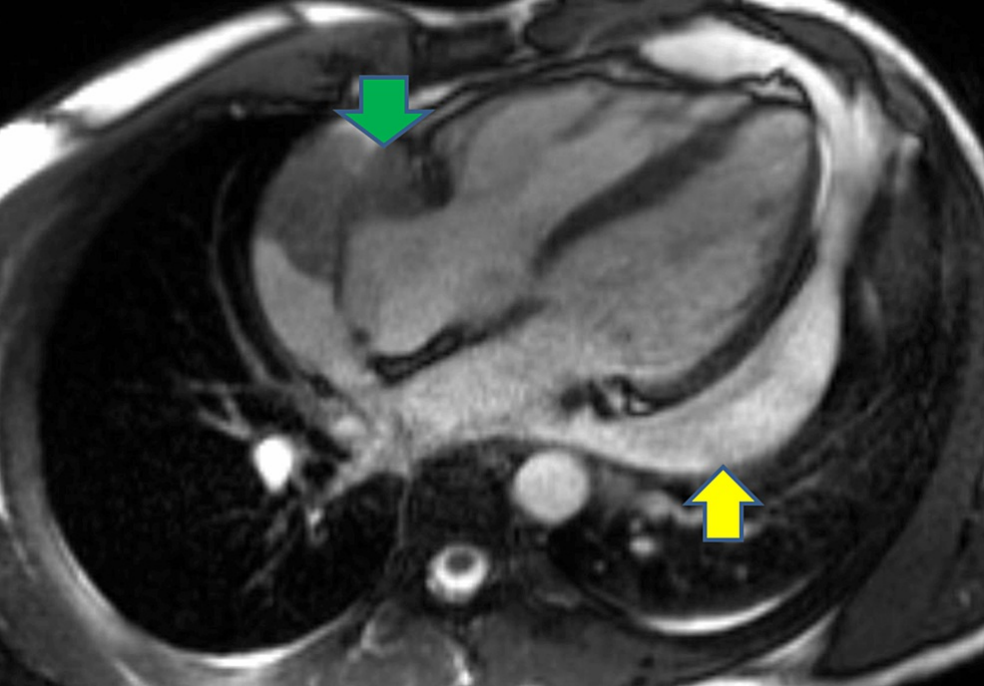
Cardiac magnetic resonance imaging showing pericardial effusion (yellow arrow) and right atrial mass (green arrow)
